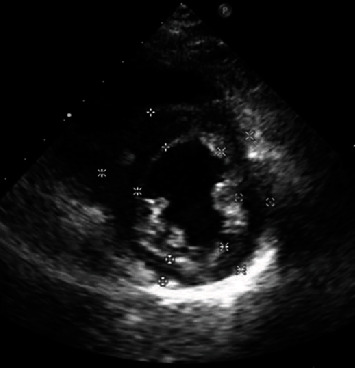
Echocardiography showing the thickened walls in the middle and lower segments.
